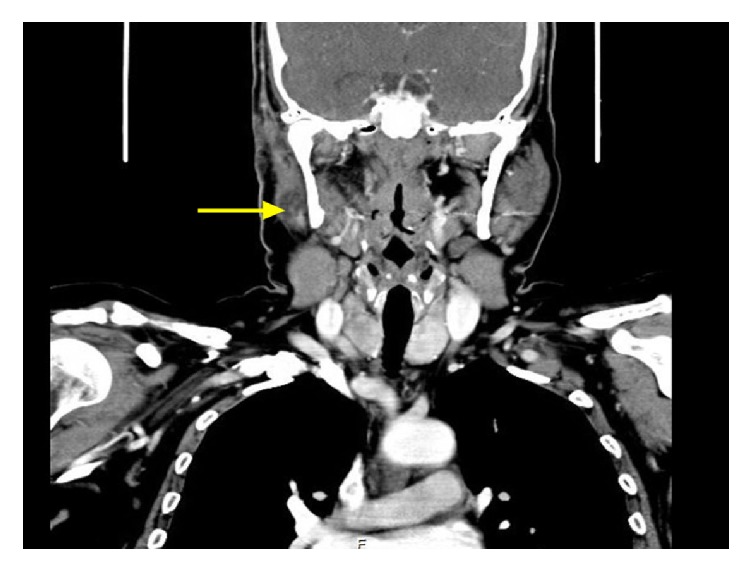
Coronal CT image demonstrates the residual parotid tumor (arrow), before the definitive surgery.
